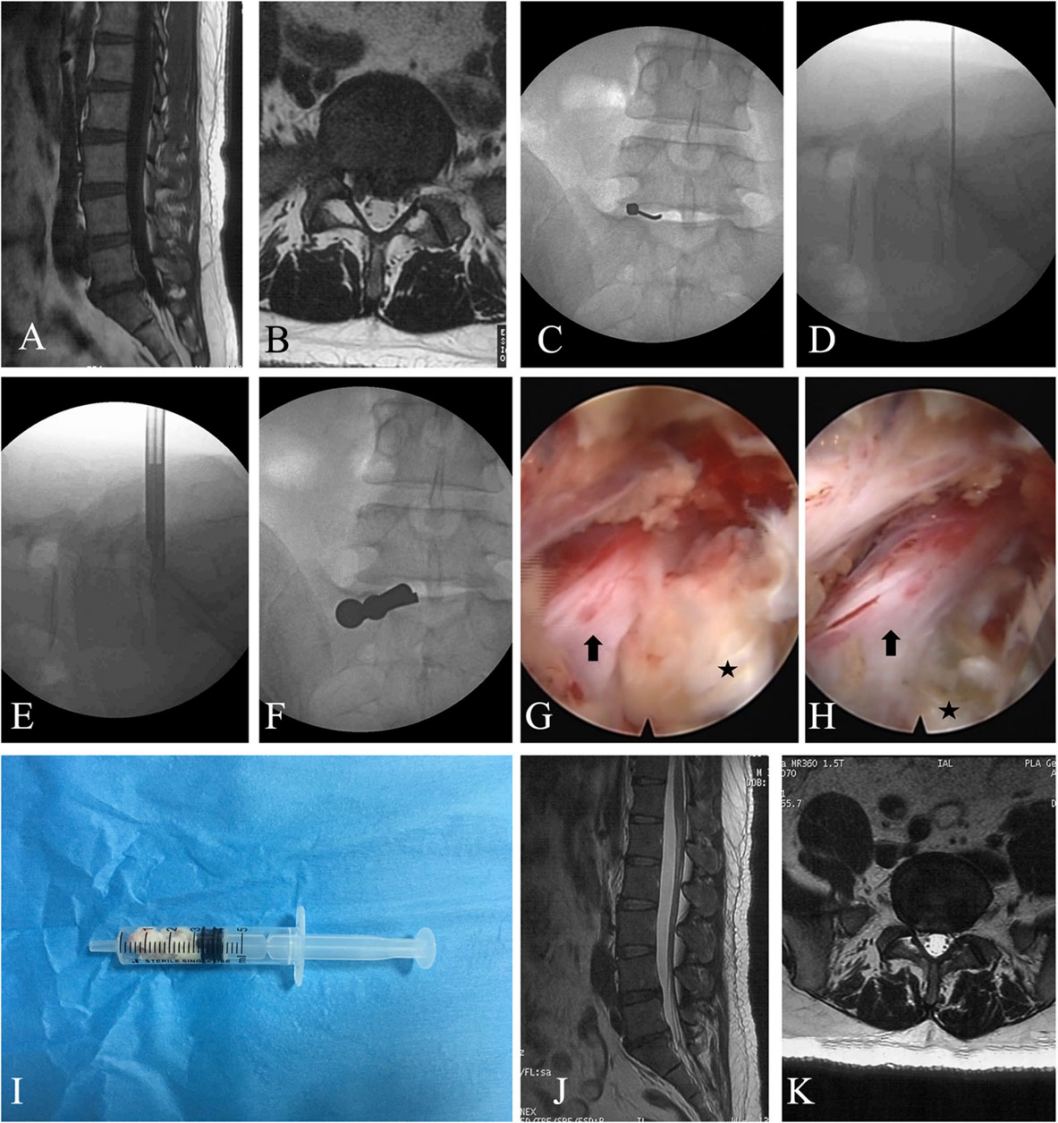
Percutaneous endoscopic interlaminar discectomy using intermittent endoscopy technique for a 40-year-old male patient with L5–S1 disc herniation. a, b Preoperative MRI image showing compression of dura and right S1 nerve root by disc herniation. c, d Place the needle into the disc. Intraoperative x-ray showing the placement of the dilator. e, f The working cannula was rotated in, and the location was confirmed with C-arm fluoroscopy before discectomy. g Intraoperative view in interlaminar access with S1 nerve root (arrow) and the herniation (star). h Intraoperative view in interlaminar access with S1 nerve root (arrow), ruptured annulus fibrosus (star) after removal of the herniation. i Disc pulposus. j, k 31 month postoperative MRI showing removal and good decompression of nerve root and dura
